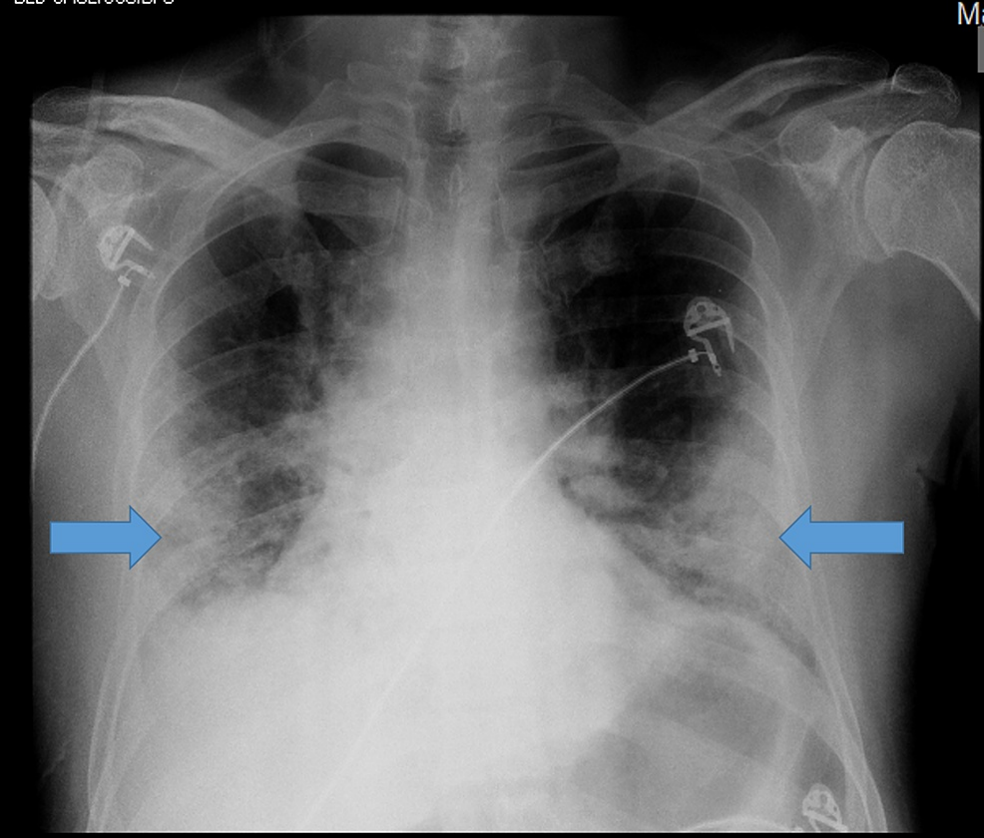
Chest radiograph showing bilateral peripheral opacities in mid and lower zones.
